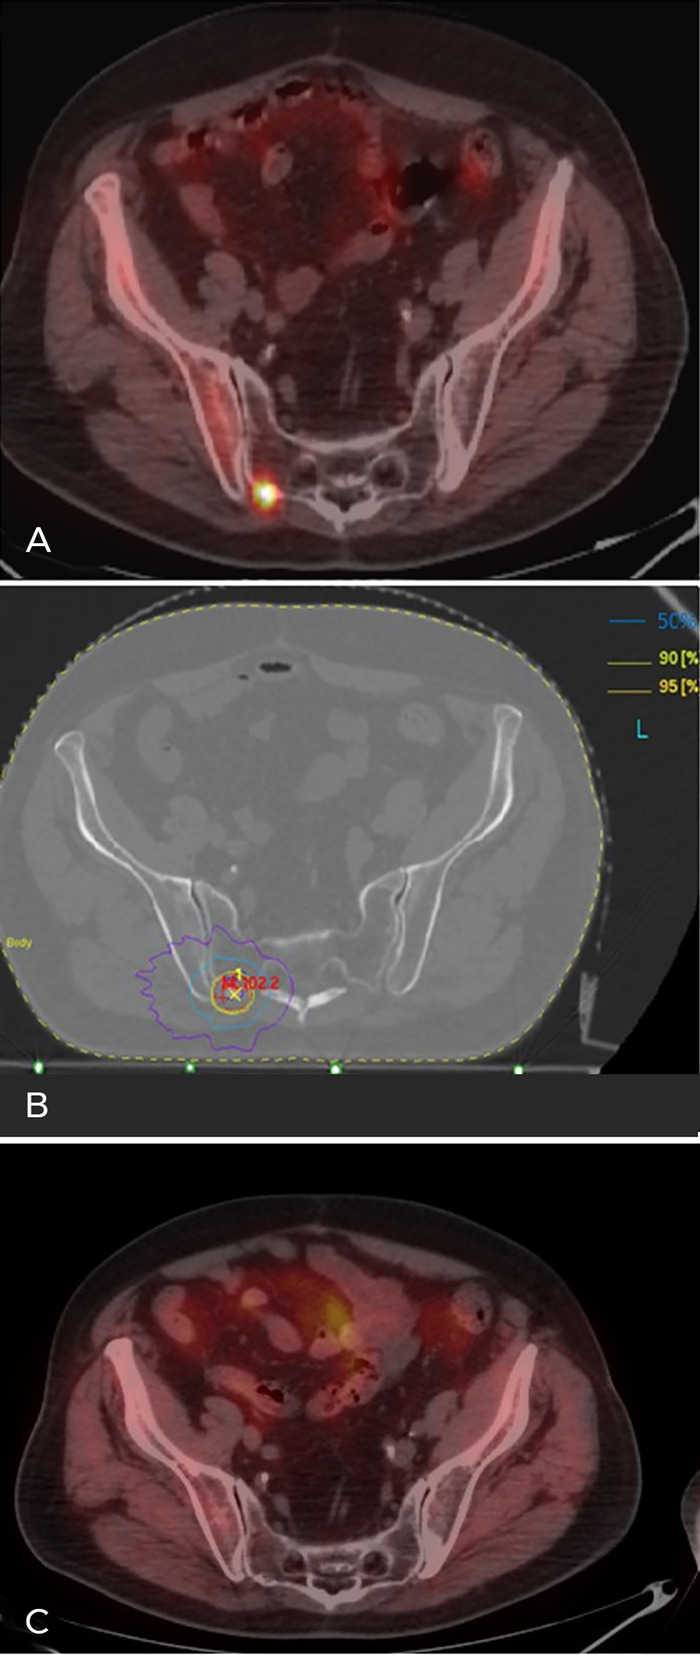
(A) F-18 choline positron emission tomography/computed tomography (PET/CT) imaging shows right sacroiliac bone metastasis corresponding to the small focus of increased radiotracer uptake. (B) Metastasis-directed stereotactic body radiotherapy was administered at 40 Gy in 5 fractions; isodoses of 95%, 90%, 50%, and 30% (yellow, green, light blue, and violet lines, respectively) indicate the small irradiated volume (isodose of 95%) and the rapid fall of dose outside the target (isodose of 30%). (C) F-18 choline PET/CT imaging showing no radiotracer uptake to the right sacroiliac bone metastasis 10 months after irradiation. (A color version of this figure is available at https://doi.org/10.31486/toj.20.0132.)
